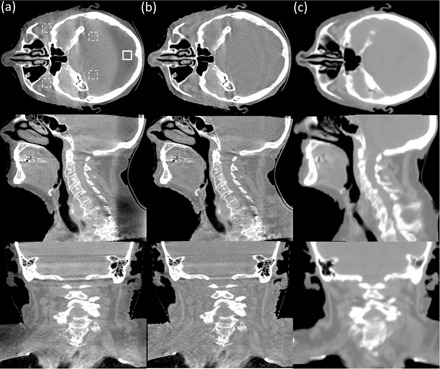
The axial, coronal, and sagittal comparison views of the head‐patient images. Display window: [−500 500] HU: (a) CBCT without correction; (b) CBCT with the proposed shading correction; (c) registered pCT. In the selected uniform soft‐tissue ROI (marked with a solid white square in (a)), the average CT numbers from (a) to (c) are −245 HU, 9 HU, and 47 HU, respectively. The SNUs calculated on the selected five ROIs (marked with solid and dashed white squares in (a)) are 18.8%, 3.0%, and 1.3%, respectively.
